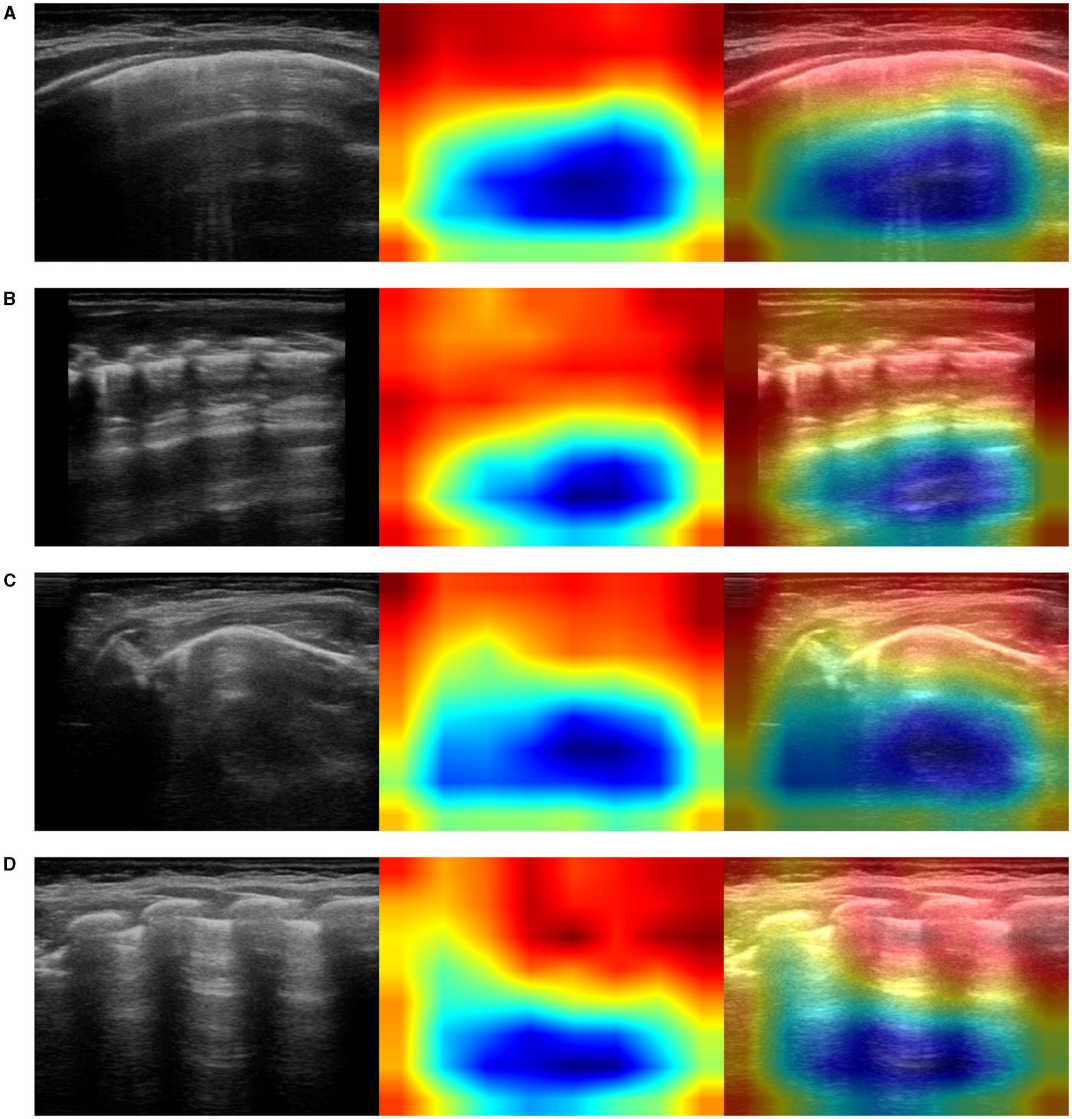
Examples of lung ultrasound images (A–D) from healthy infants correctly predicted by the best performance model (Inception-v3). Left: original LUS image; middle: class activation mapping produced by the Grad-CAM visualization; right: the class activation mapping overlaid transparently on the original LUS image. Note that red and orange regions correspond to high scores for the predicted class and correctly highlight diagnostic-relevant features: regular pleural lines; presence of short vertical artifacts; presence of long vertical artifacts single and/or multiple, non-confluent and/or confluent, with possible uneven distribution and/or involving multiple lung areas with a prevalence of the right and/or left hemithorax, depending on the gestational age and the current age of the patient.
